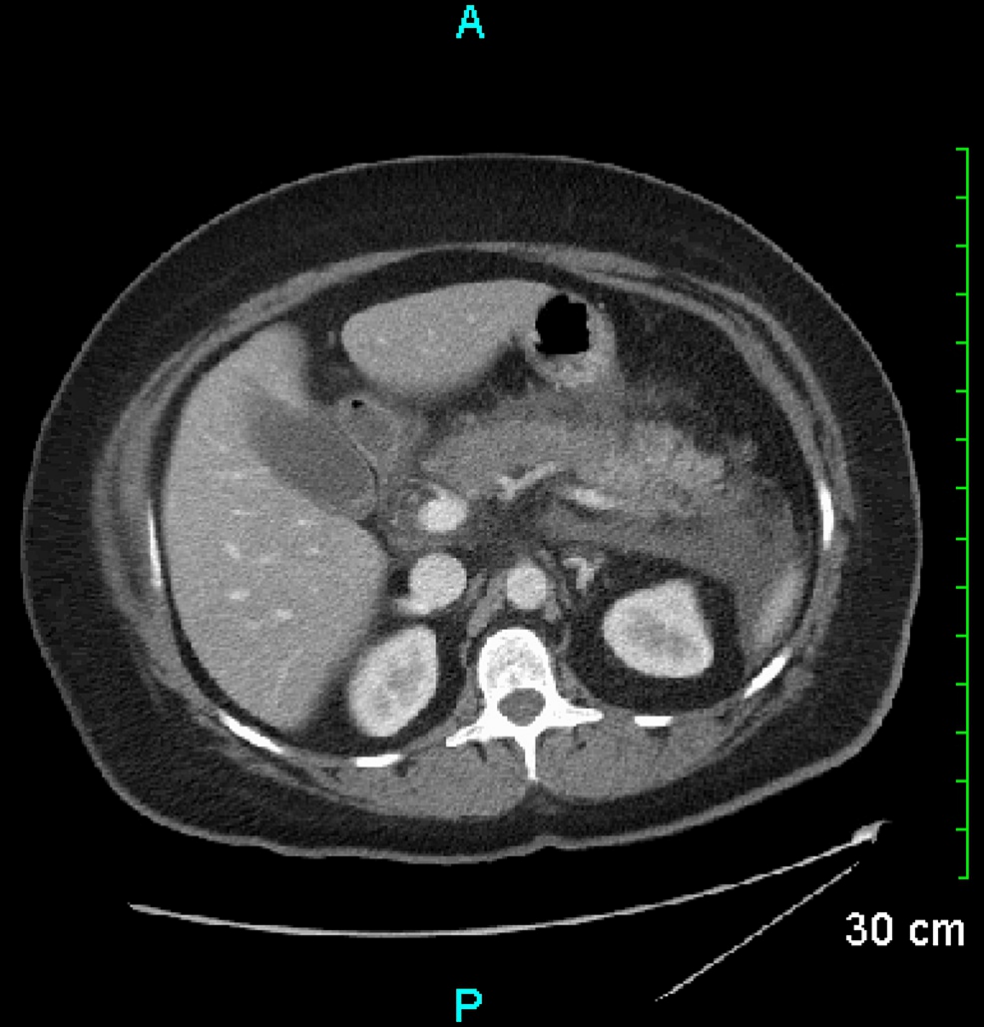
CT abdomen with IV contrast upon admission. Decreased attenuation of the pancreatic body with fat stranding consistent with acute pancreatitis. Gallstones are likewise seen.
